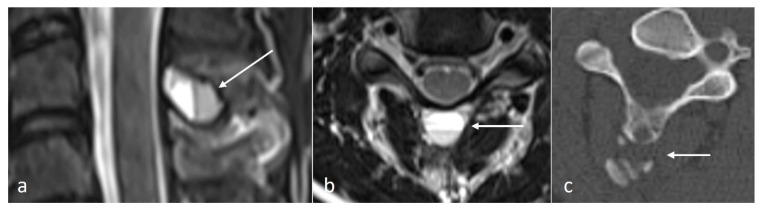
Sagittal T2 (a), axial T2 (b) and axial CT (c) images demonstrating an aneurysmal bone cyst of a cervical spinous process (white arrows). Note the lytic osseous destruction on CT and the characteristic presence of fluid–fluid levels on MRI.
